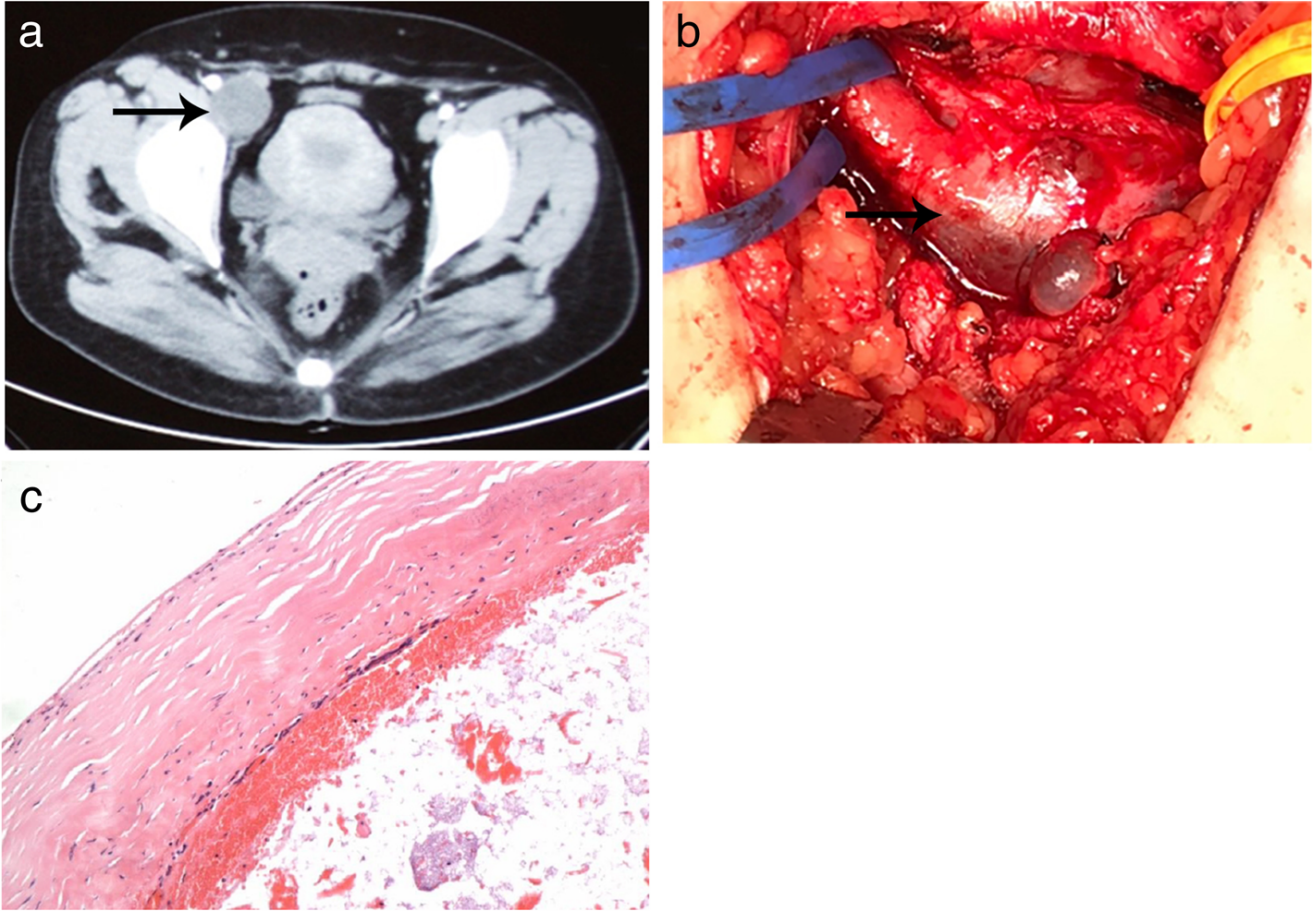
Image data and pathology result for patient NO.1. a. A CT showed a cystic space-occupying lesion (black arrow) in the inguinal region. b. At surgery, the synovial cyst (black arrow) was observed directly communicating with the hip joint. c. The pathology result confirmed a synovial cyst
